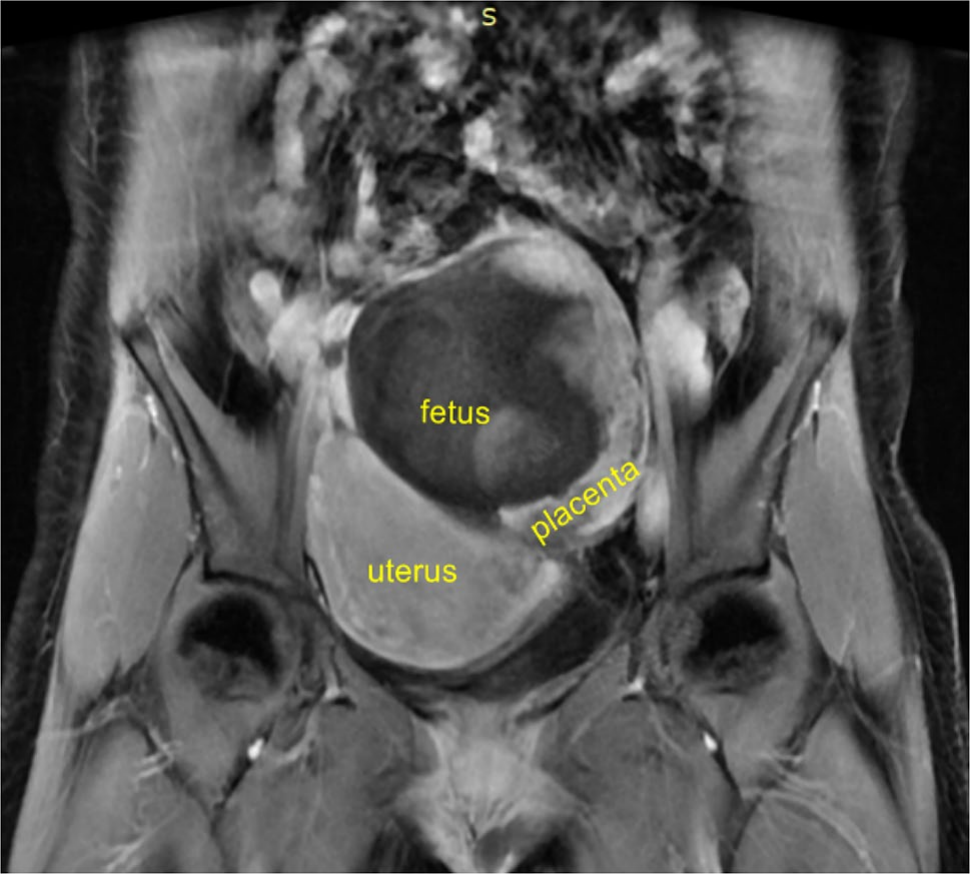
Abdominal pregnancy: MRI coronal position. The fetus is located at the left rear side of the uterus
